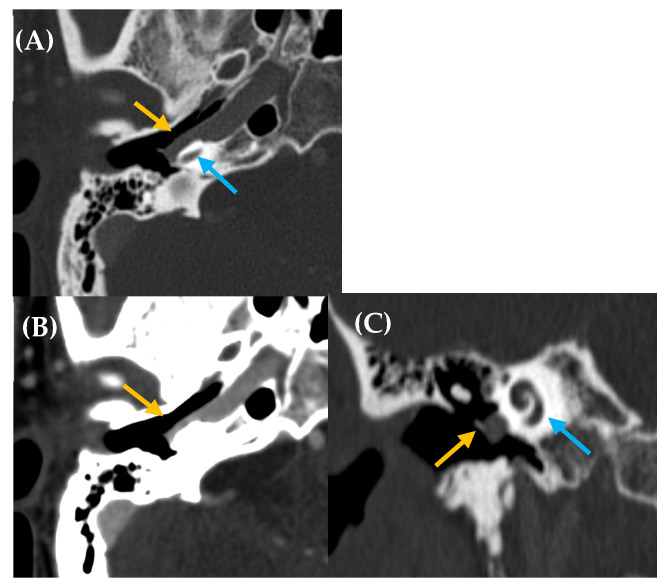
Aberrant intratympanic course of the right internal carotid artery, which is in contact with the cochlea (yellow arrow: aberrant ICA; blue arrow: cochlea). A 33-year-old man with right-sided PT of several years’ duration. Otoscopy revealed a pulsatile tumor in the right ear. CT imaging (A), axial plane bone window; (B), axial plane, contrast enhanced with soft tissue window; and (C), MPR coronal plane reconstruction) demonstrated a space-occupying lesion with a tubular appearance in the right mesotympanum, adjacent to the cochlear promontory, along with an enlarged inferior tympanic canaliculus and absence of the carotidotympanic bone septum.
